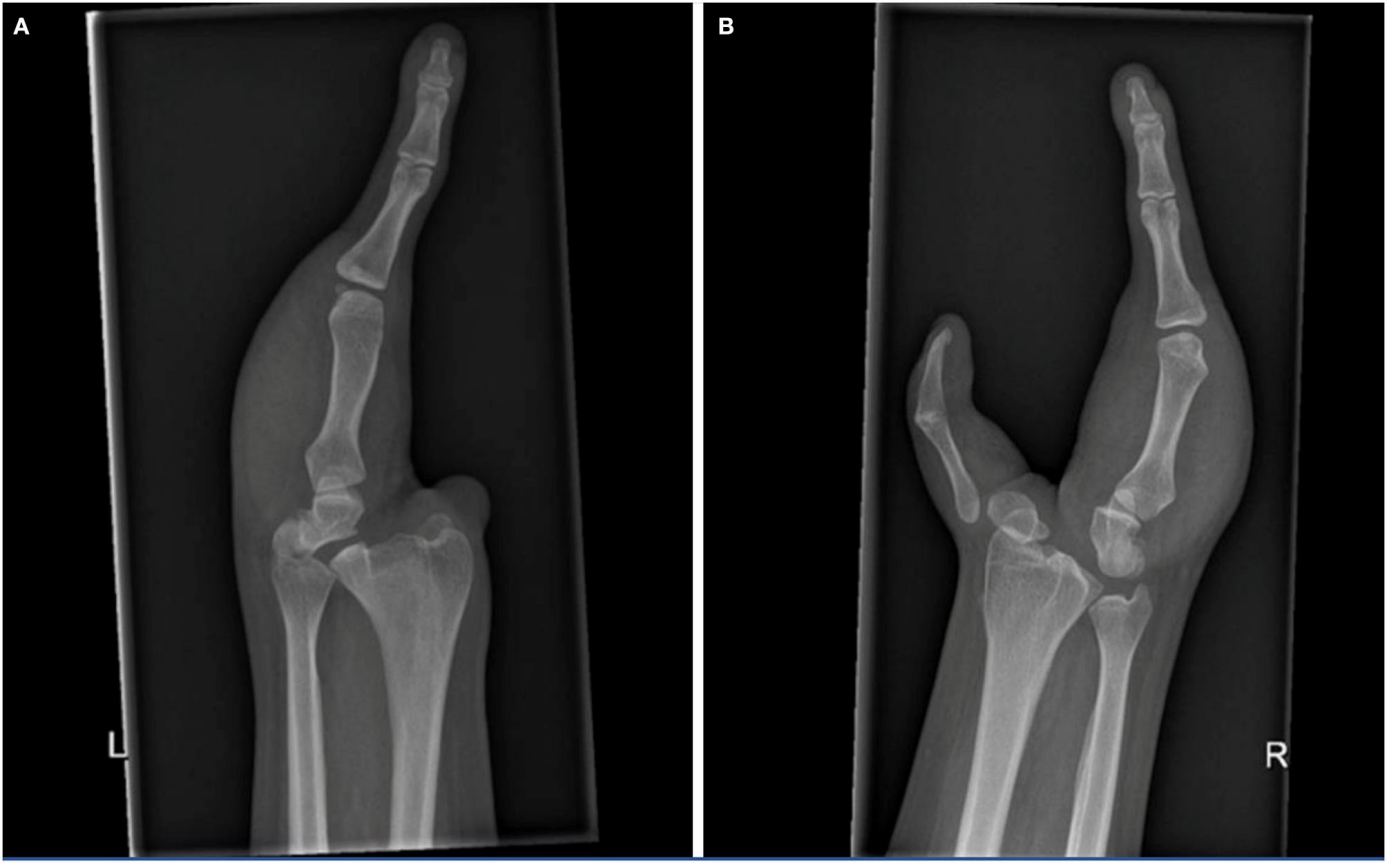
X-rays of the left (A) and right hand (B), show the osseous structures in this patient with cleft hands. Note the symphalangism of the right thumb bones.
